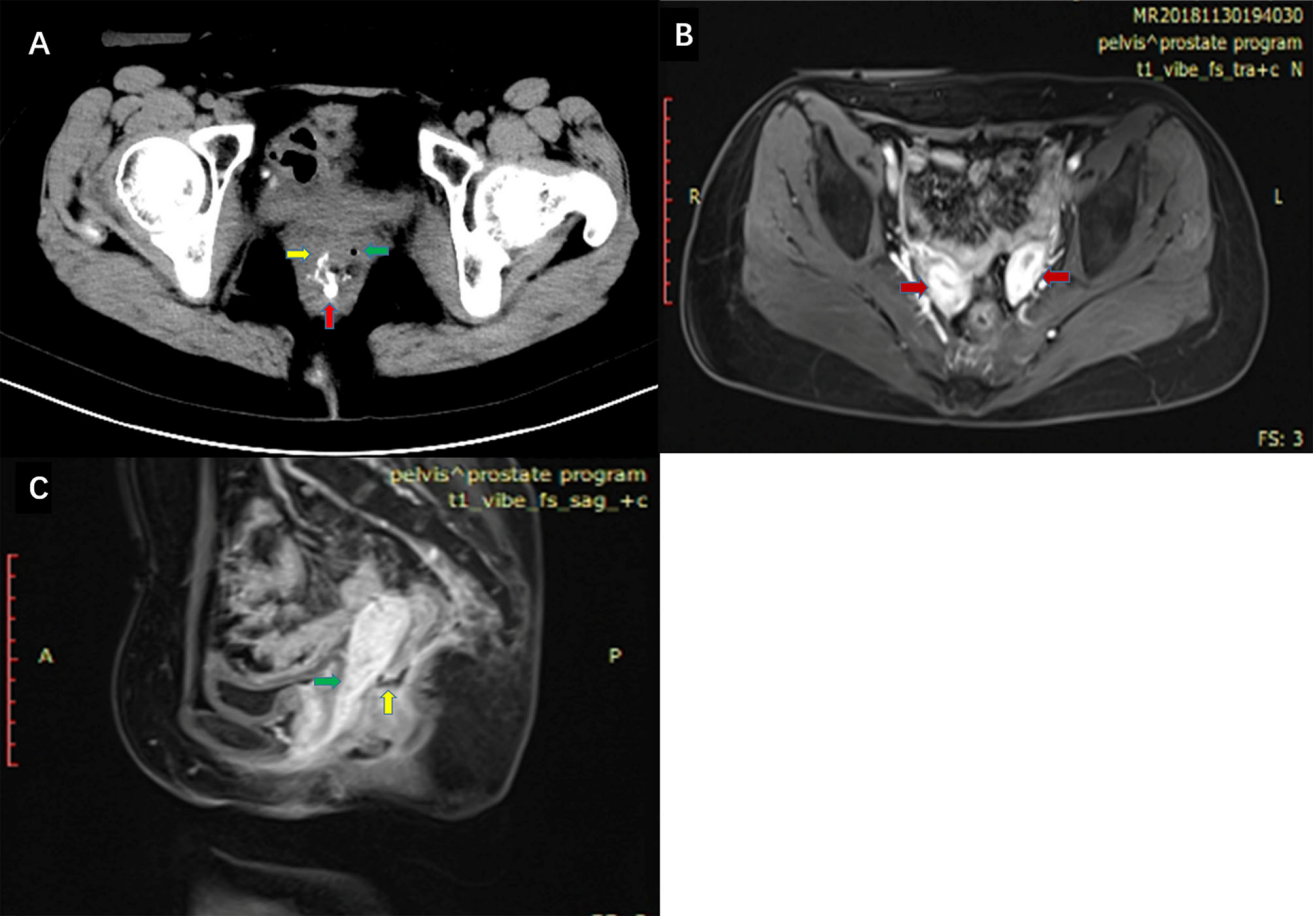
(A) High density contrast flow from the rectum into the right vagina in pelvic CT scans after barium enema. Red arrow: rectum. Green arrow: left vagina. Yellow arrow: right vagina. (B) Enhanced pelvic MRI. Red arrow: double uterine. (C) Enhanced pelvic MRI. Green arrow: vagina. Yellow arrow: fistula between the rectum and vagina.
